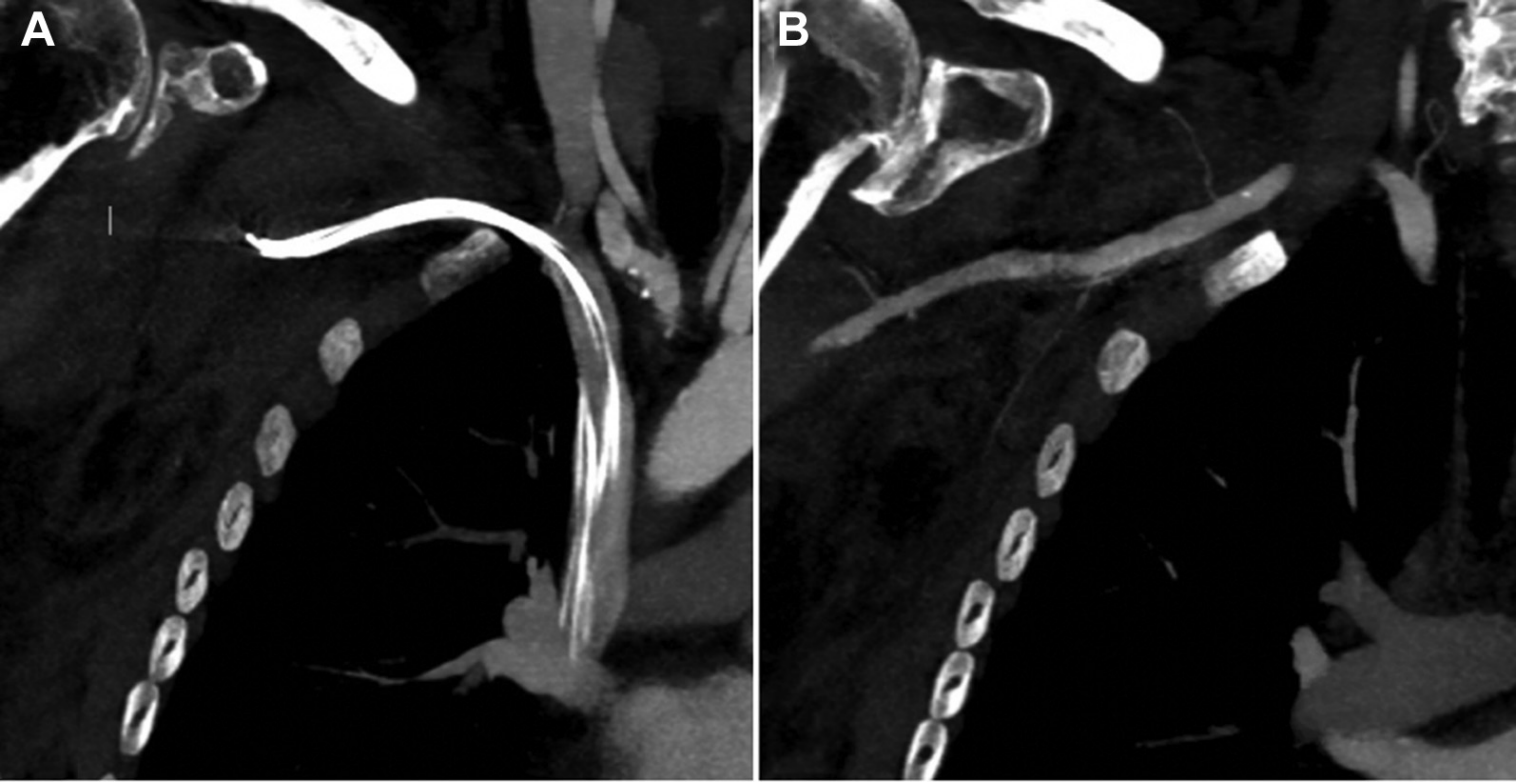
Computed tomography scan of the chest in the coronal view revealing (A) biventricular implantable cardiac defibrillator leads entering the axillary/subclavian vein and (B) active bleeding from the pectoral branch of the thoracoacromial artery.
